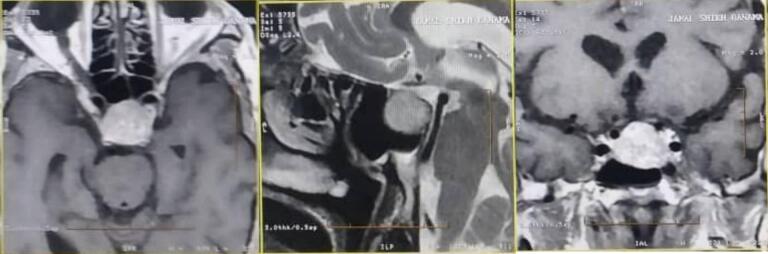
Brain MRI (horizontal, sagittal, and coronal planes) showing pituitary’ tumor.
